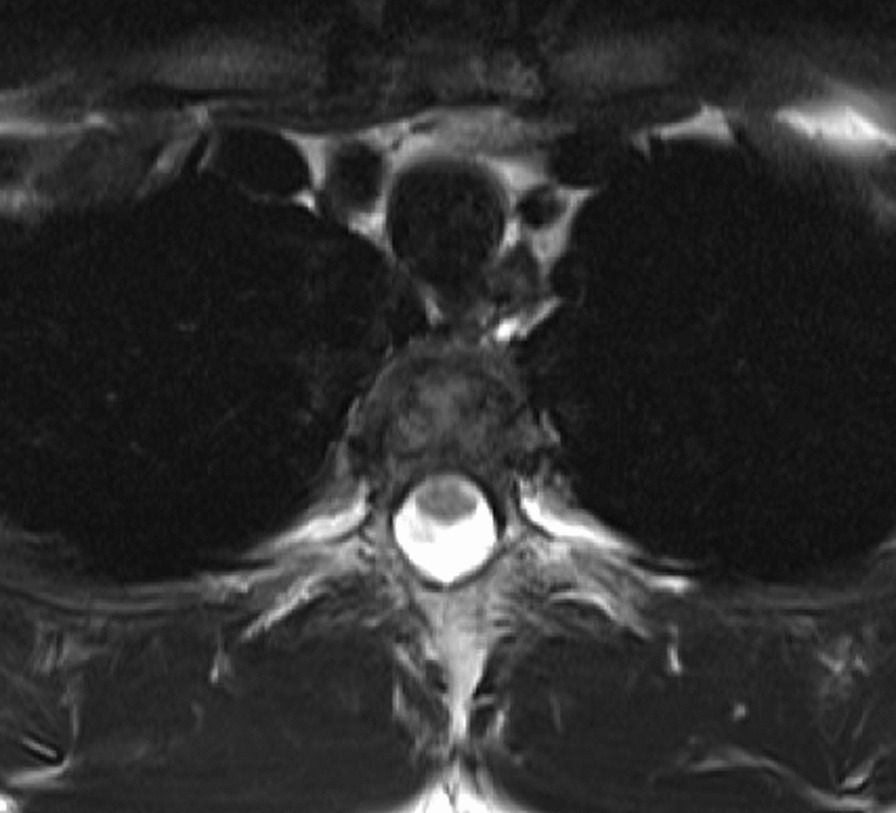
Transverse MRI (T2-weighted) at 4-months post-surgery. MRI findings reveal improved spinal fluid loss in the anterior spinal cord, in the Th4–5 region
